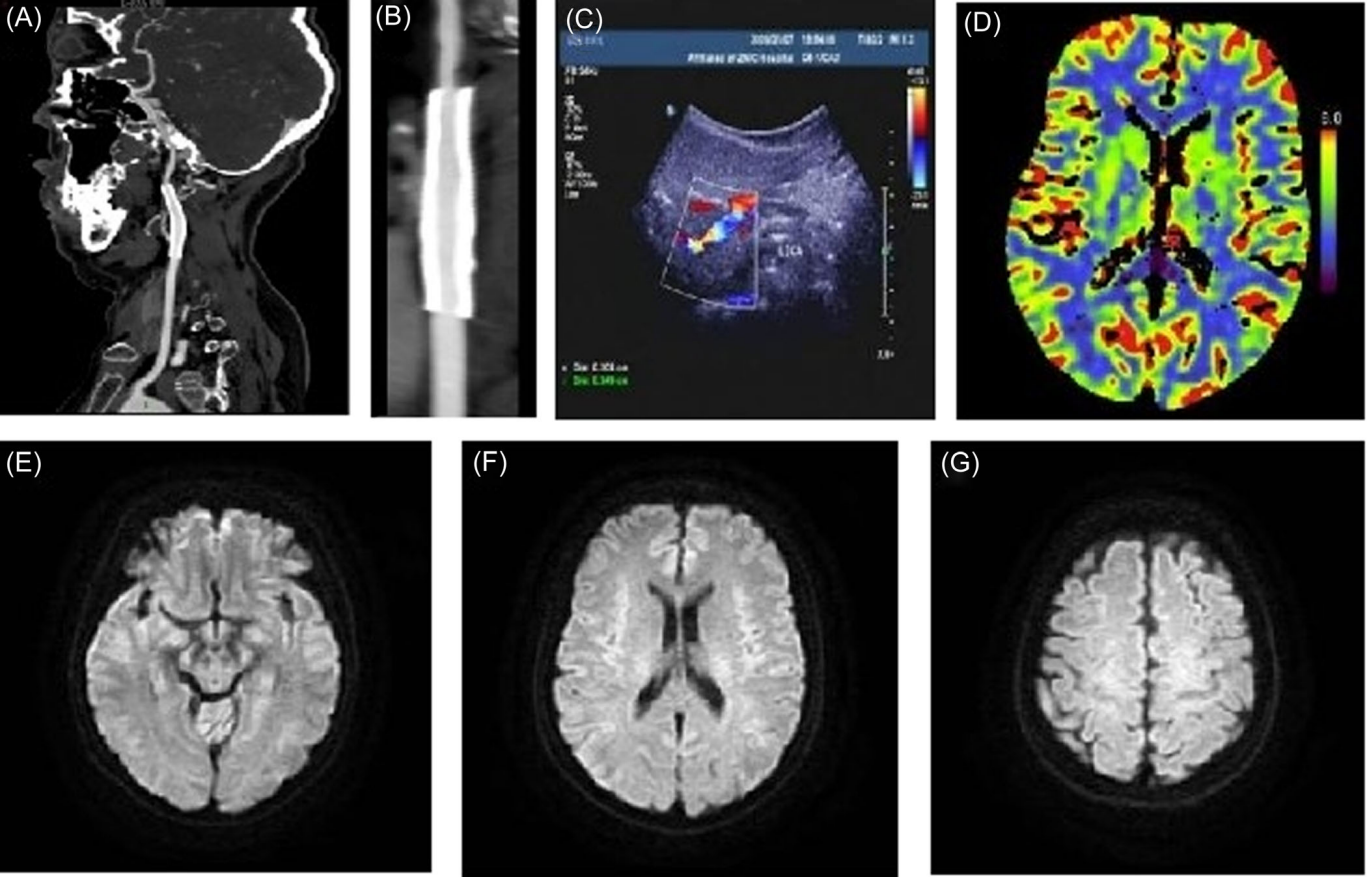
(A, B) Carotid artery computed tomography (CT) angiography. (C) Carotid artery ultrasound showing unobstructed blood flow in the stent. (D) Cranial CT perfusion imaging showing normal cerebral perfusion in both hemispheres. (E–G) Brain magnetic resonance imaging showing no infarction in the left occipital lobe and parietal lobe. [Color figure can be viewed at wileyonlinelibrary.com]
